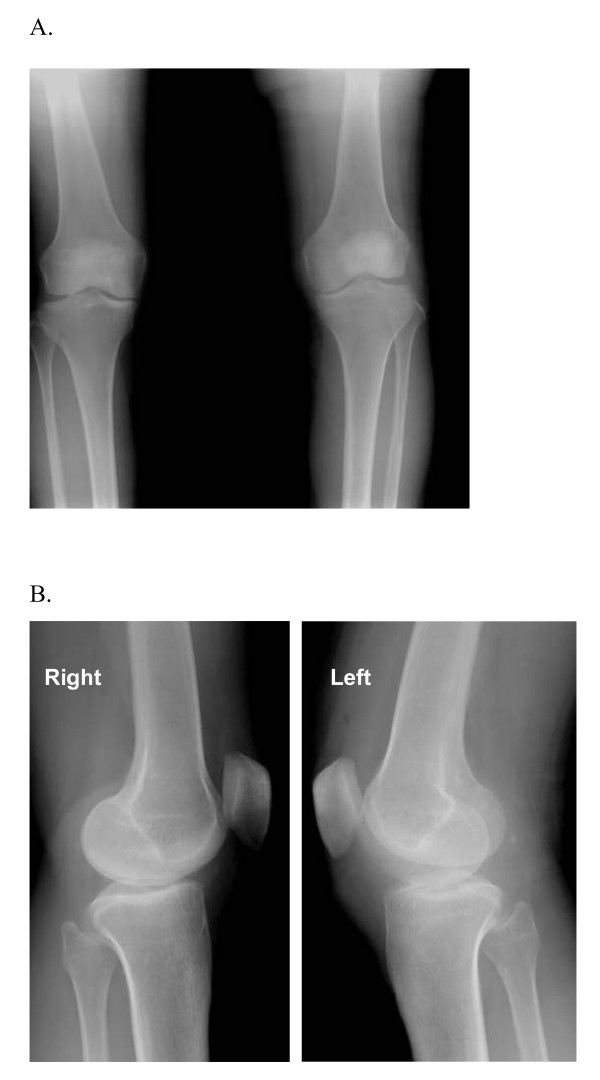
Anteroposterior and lateral standing radiographs of the both knee. (A) Anteroposterior and (B) lateral (right and left) radiographs showing bilateral widening of lateral joint spaces, and increased concavity and subchondral sclerotic of the medial tibial plateau.
